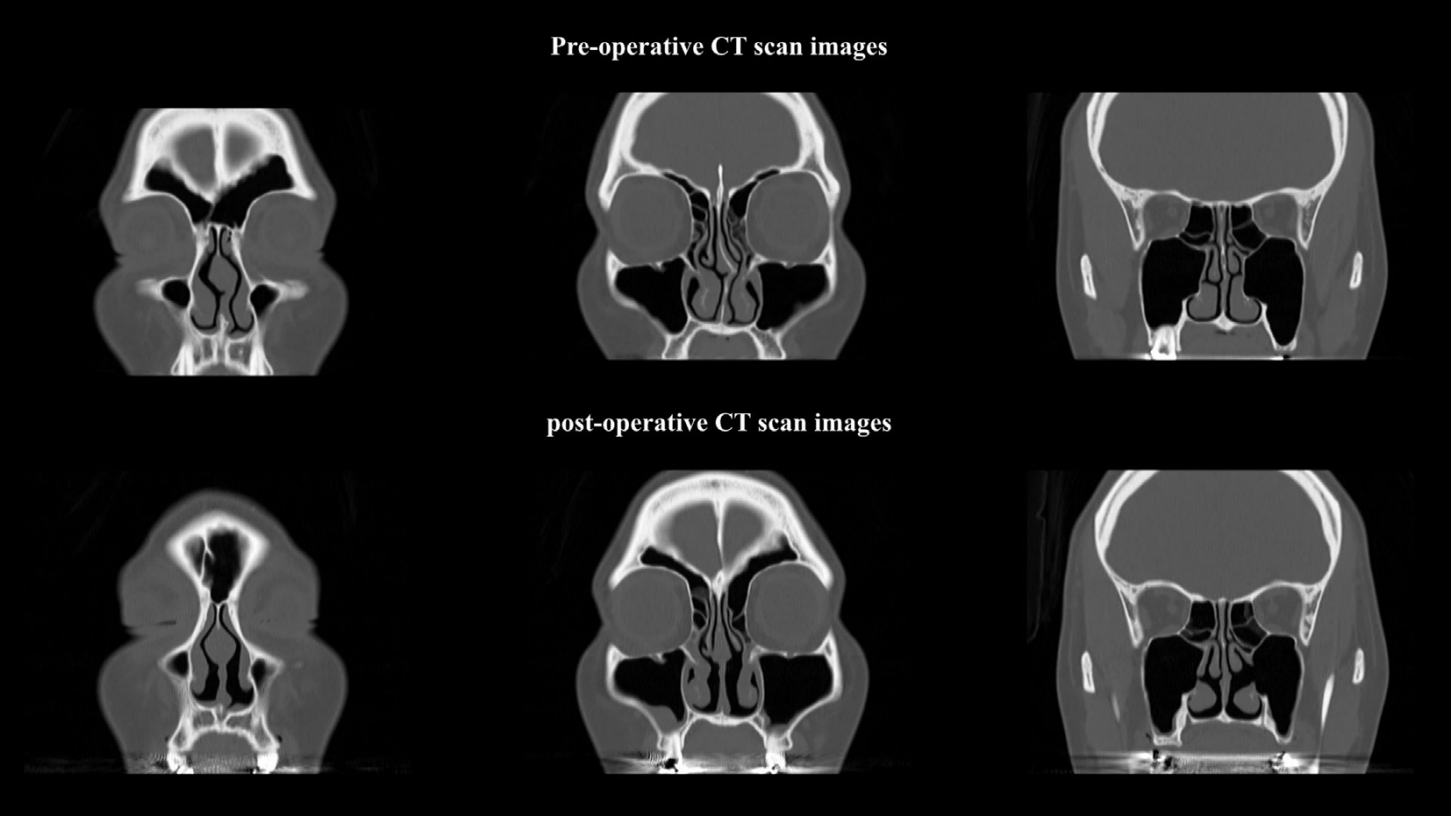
Post-operative computed tomographic images show an expanded bilateral common nasal meatus.
